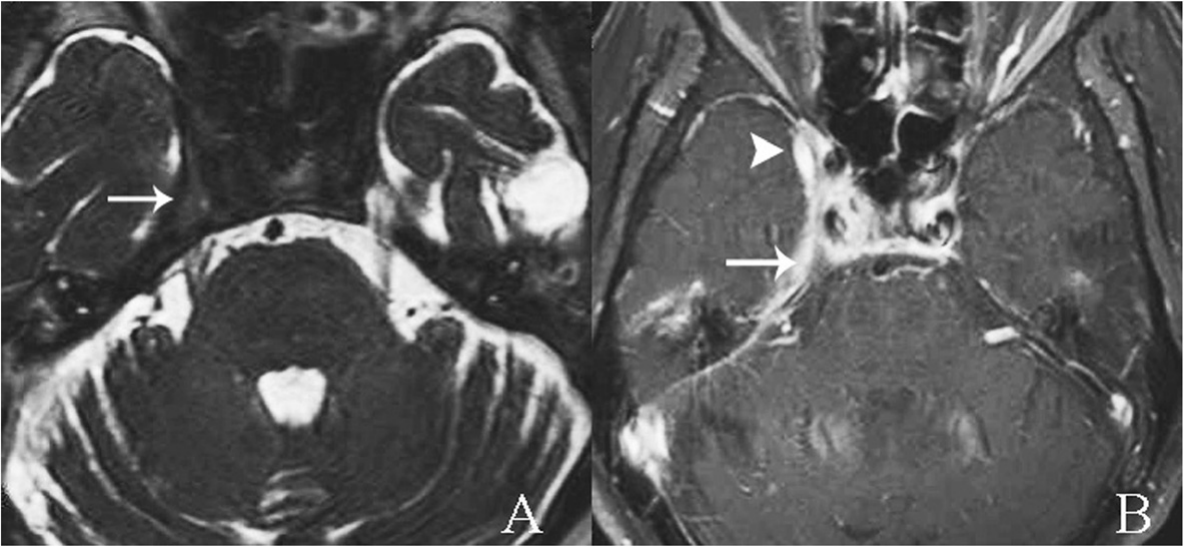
Axial magnetic resonance imaging at the level of the cavernous sinuses. A. Thin-slice axial T2-weighted images obtained by three-dimensional driven equilibrium sequence revealed edematous right trigeminal ganglion (arrow). B. Postgadolinium-pentetic acid spin echo T1-weighted with fat suppression magnetic resonance imaging of temporal bone. Edematous right trigeminal ganglion within right Meckel’s cave (arrow) surrounded by abnormal prominent enhancement in right Meckel’s cave and cavernous sinus (arrowhead).
